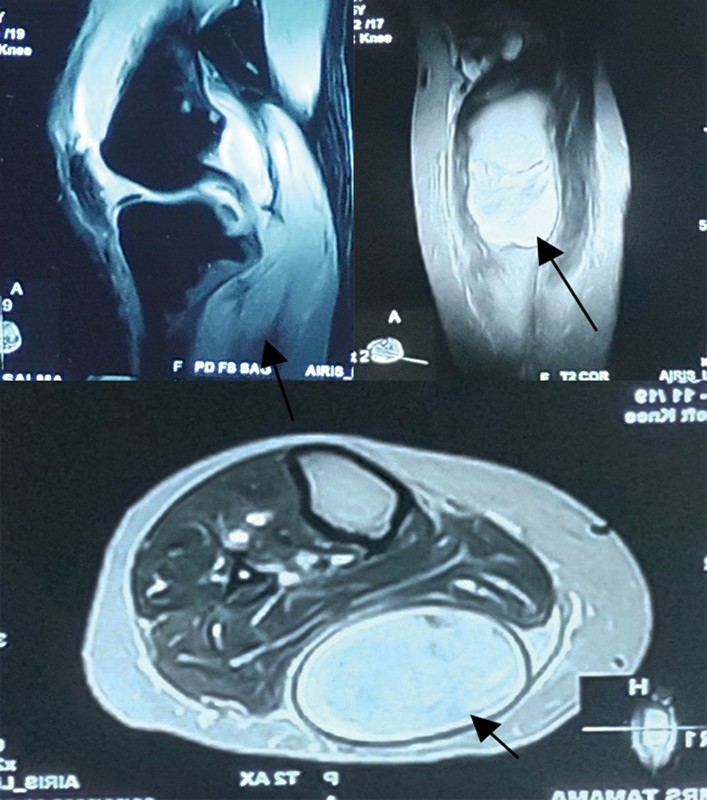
Magnetic resonance imaging (MRI) scan of the knee joint sequences showing a complex, multiloculated cyst with an internal hemorrhagic component arising from the posteromedial aspect of the knee joint and extending inferiorly into the calf region, causing compression over the posterior muscular compartment (upper left: sagittal section; right: coronal view; and bottom: axial view); all sections are slices > 2 mm, and T2-weighted images are shown, with all arrows pointing toward lesions in different views.
